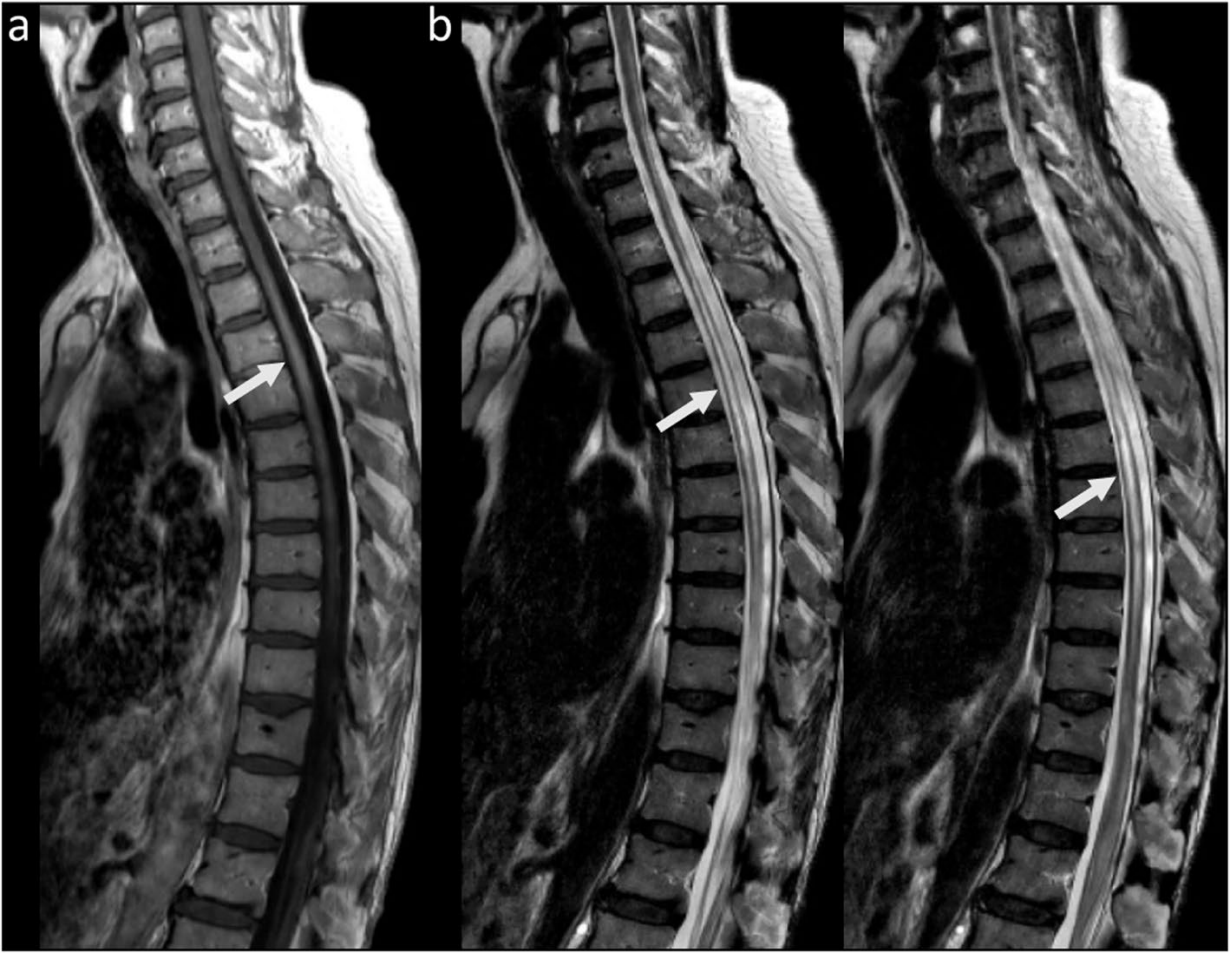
Spinal cord MRI (a) Sagittal T1-weighted + GD image; white arrow indicates GD-enhancement ranging from C3 to C5 (b) Sagittal T2-weighted images; white arrows indicate T2-signal alteration ranging from C4 to T10
